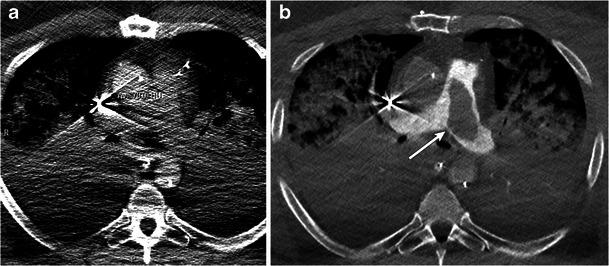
Pulmonary embolism. Axial CT image of a 44-year-old male on central VA ECMO a shows insufficient opacification of the pulmonary arteries using the standard protocol secondary to siphoning of IV contrast by ECMO. Diagnositc images are obtained in the same patient b after putting the circuit in a minimal flow state (500 ml/min) for 15 s with reinjection of IV contrast. Thrombus is seen in the main pulmonary artery (b, arrow)
